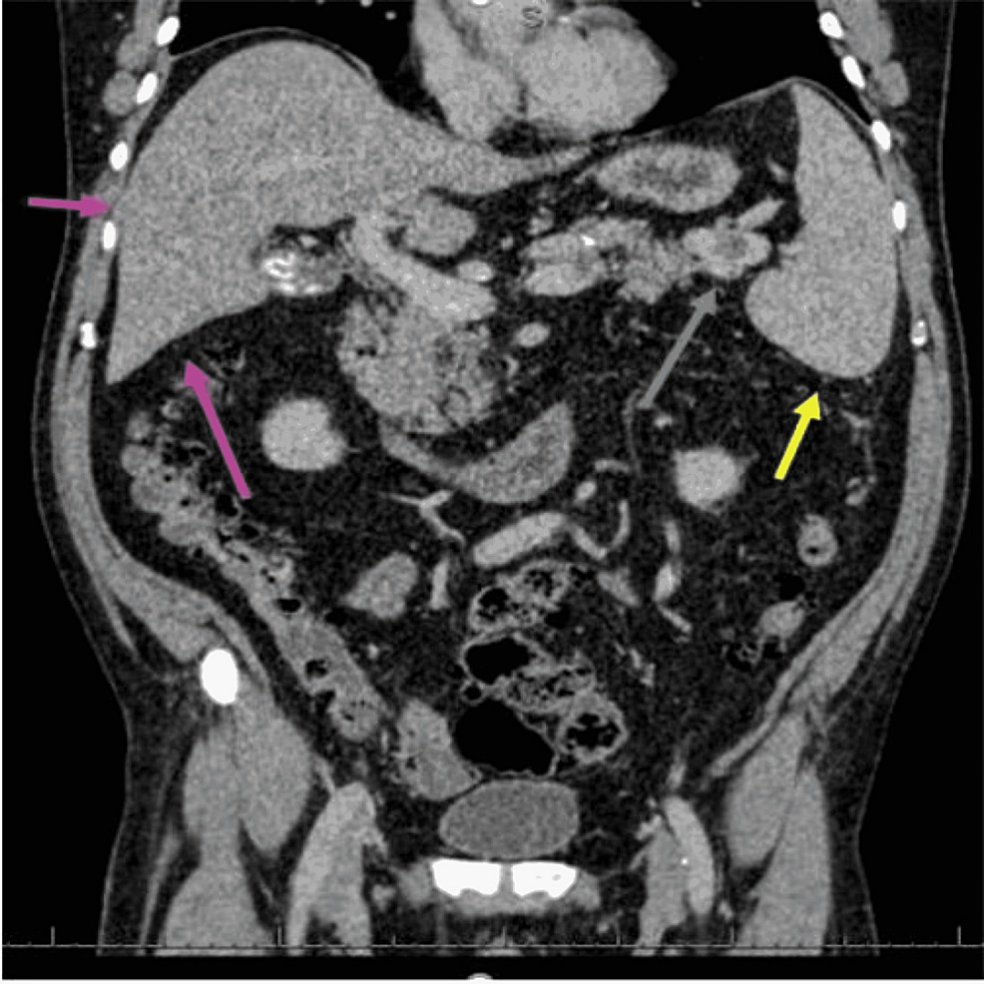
Computed tomography (sagittal view) with the irregular contour of the liver (pink arrows), partially occluded splenic vein thrombus (blue arrow), and splenomegaly (yellow arrow).
